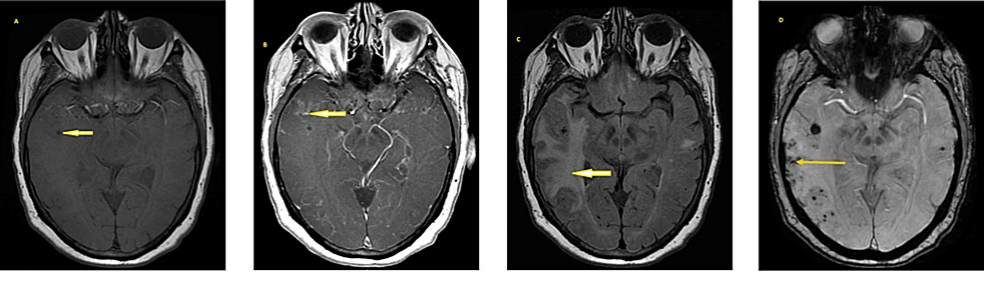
MRI brain (A) T1 pre-contrast, (B) T1 post-contrast, (C) T2 flair, (D) SWAN
